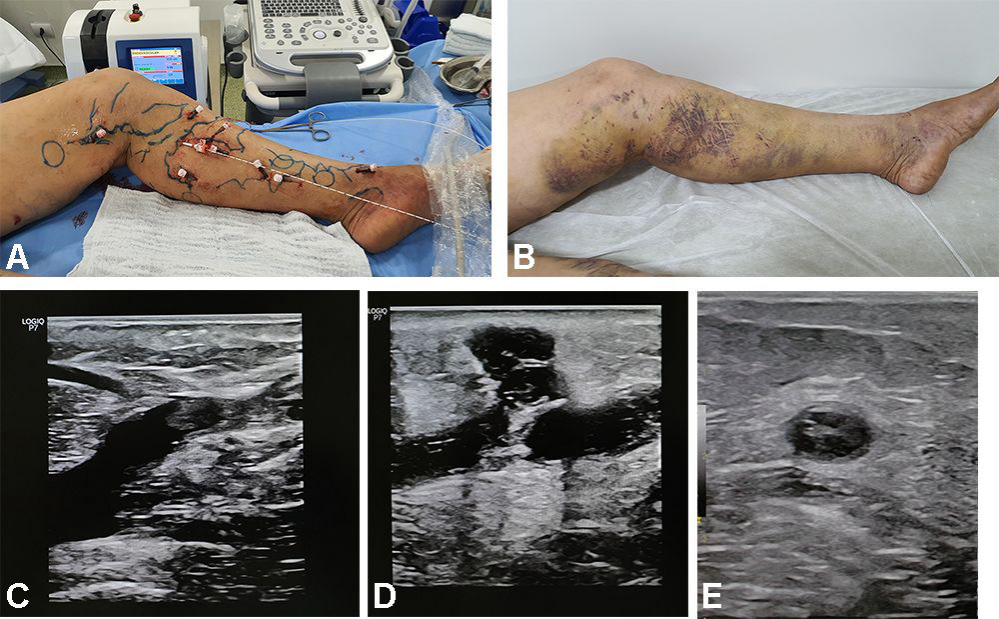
Ultrasound findings at 7 days. (A) Perioperative image showing the multiple punctures and catheters; (B) Postoperative appearance at 7 days; (C) Ultrasound image of the saphenofemoral junction close to the puncture site on the 7th postoperative day; (D) Ultrasound image of the great saphenous vein close to the puncture site on the 7th postoperative day; (E) Transverse view of the great saphenous vein in the thigh on the 7th postoperative day.
